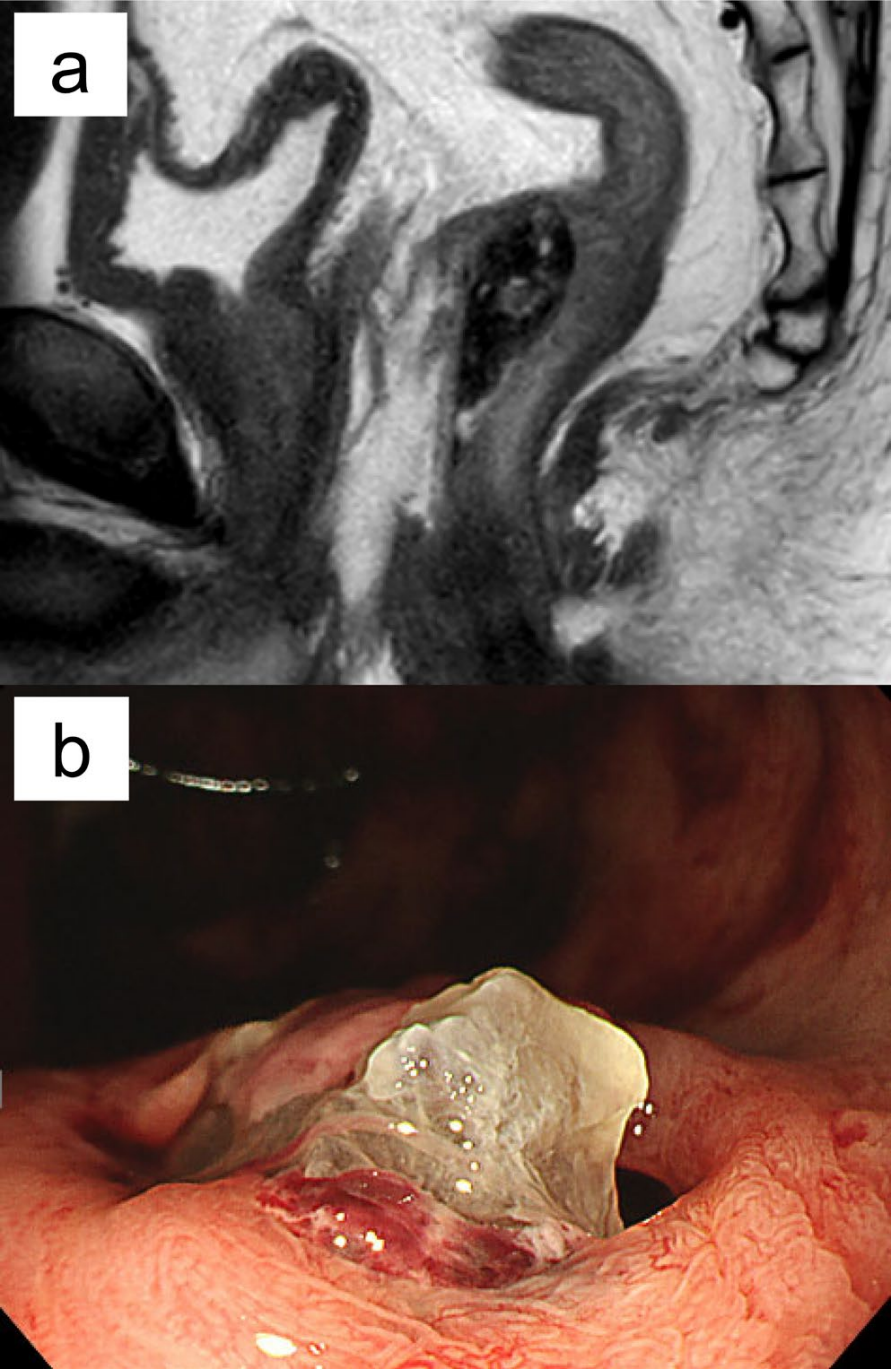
(a) Sagittal T2‐weighted MRI showing hydrogel spacer migration into the anterior rectal wall. (b) Colonoscopy image showing the spacer protrudes from the base of the ulcer, forming a fistula.
